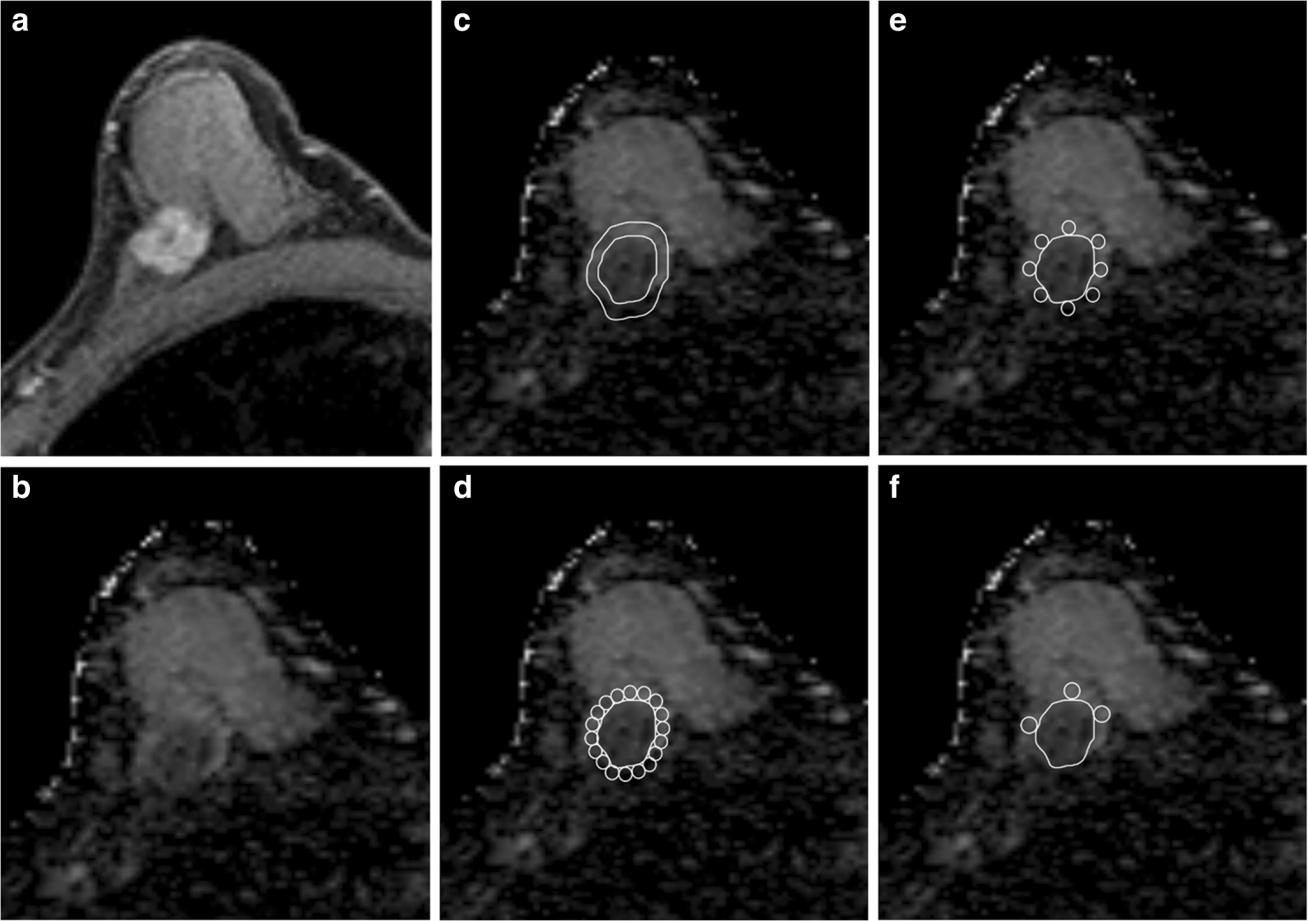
Methods to measure peritumoral ADC values in a regular-shaped oval tumor. a T1-weighted gadolinium-enhanced thin-slice source image showing the oval mass lesion with parenchymal background. b Apparent diffusion coefficient (ADC) map corresponding to the mass lesion. c Method 1 (M1): band ROI method, a band ROI generated adjacent to the tumor border on ADC to cover the whole tumor’s surrounding area. d Method 2 (M2): whole tumor surrounding ROI method, circular ROIs placed next to each other adjacent to the tumor border to cover the whole tumor’s surrounding area. e Method 3 (M3): clockwise ROI method, 8 circular ROIs placed clockwise at the 0:00, 1:30, 3:00, 4:30, 6:00, 7:30, 9:00, and 10:30 o’clock positions. f Method 4 (M4): visual assessment ROI method, 3 circular ROIs placed according to the visually perceived most increased ADC areas
